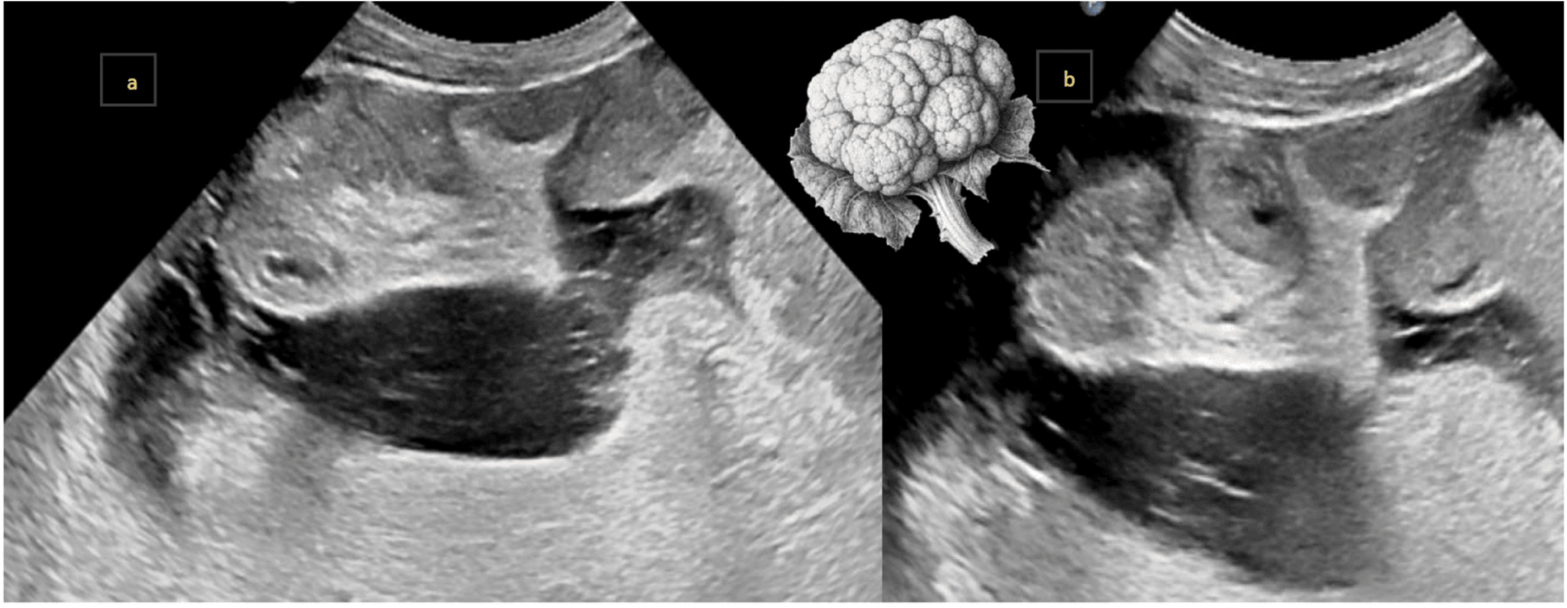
a and b: Abdominal ultrasound images showing central clumping of small bowel loops toward the root of the mesentery, resembling a cauliflower with a trilaminar appearance: hyperechoic membrane, hypoechoic bowel wall, and hyperechoic lumen.
